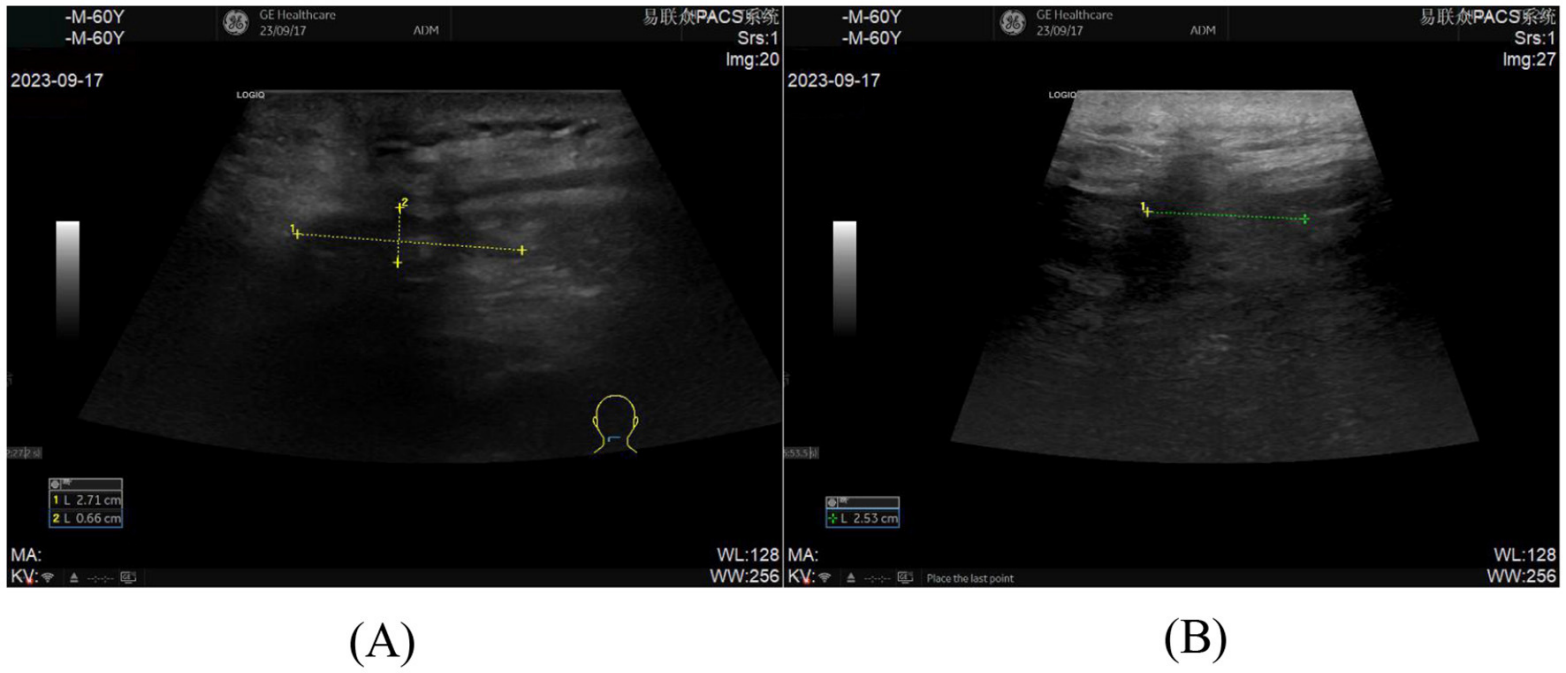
(A, B) Follow-up ultrasonography on postoperative day 7 after ultrasound guided catheter drainage demonstrates a residual anechoic fluid collection measuring approximately 2.71 cm × 0.66 cm × 2.53 cm.
